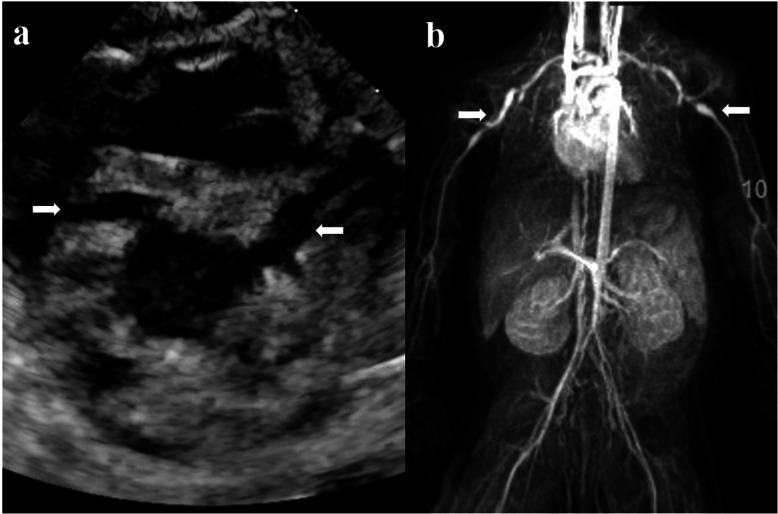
a Echocardiography at 2 weeks after the onset of KD revealed medium coronary artery aneurysms (arrow) in both the left and right coronary arteries. b Magnetic resonance angiography at 2 weeks after the onset of KD revealed bilateral axillary artery aneurysms (arrow)
